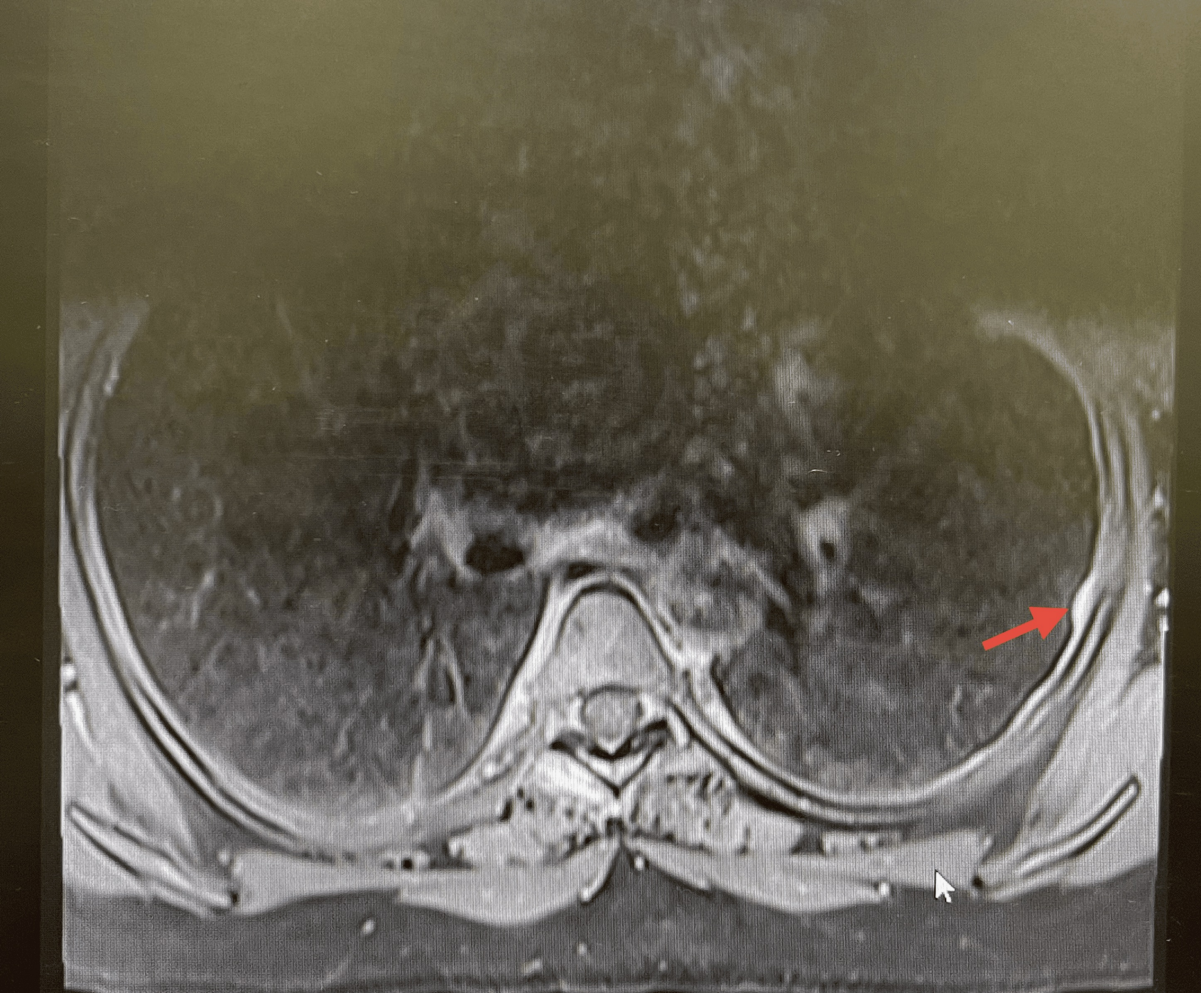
MRI showing focal left-sided pleural thickening (arrow), contributing to initial suspicion of metastatic spread.
